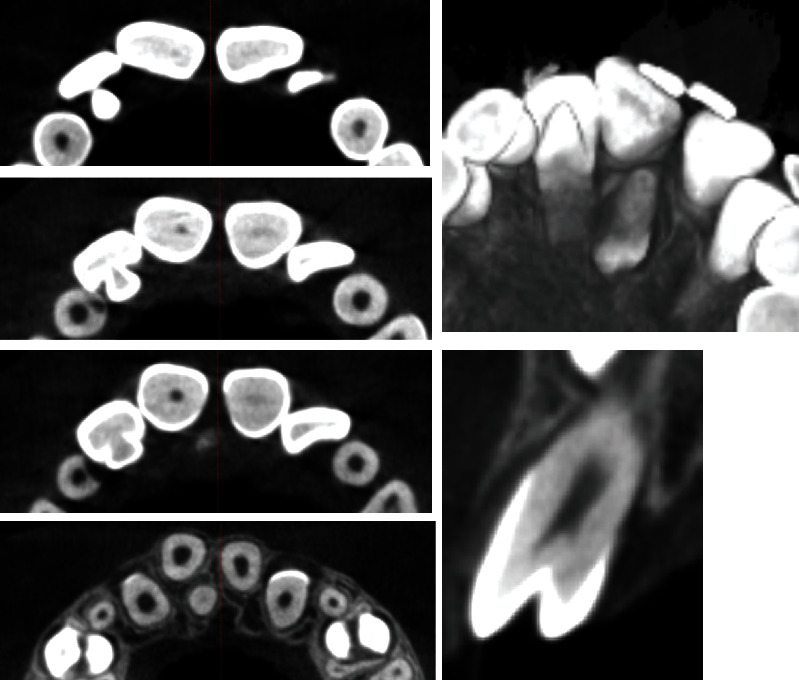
CBCT examination and transverse sections.
